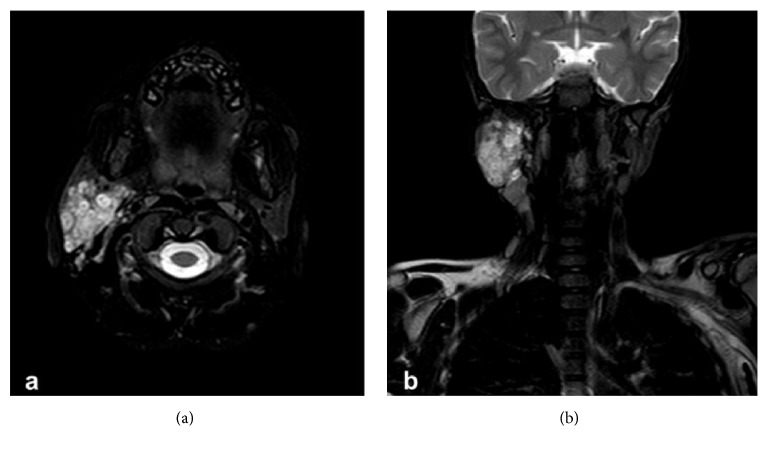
Axial (a) and coronal (b) fat suppressed T2-weighted MR images indicating a large, heterogeneous, lobulated, fusiform, and hyperintense mass in the right parotid region. The multinodular lesion exhibits a target-like appearance due to a peripheral zone of higher intensity. Note the enlarged jugular and spinal accessory lymph nodes.
